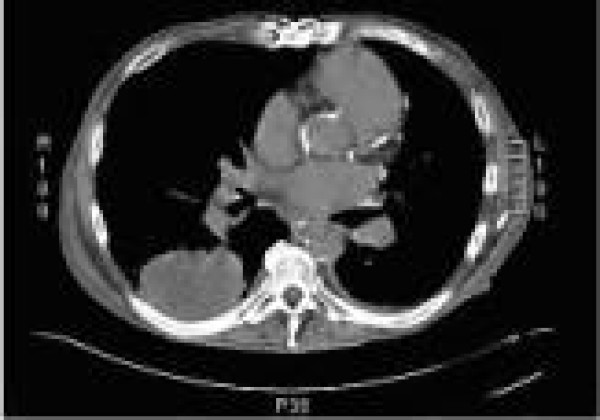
CT chest shows pleural based lesion in the right lower lobe measuring 7 cm (SI) × 5.4 cm (AP) × 7.9 cm (TV).
